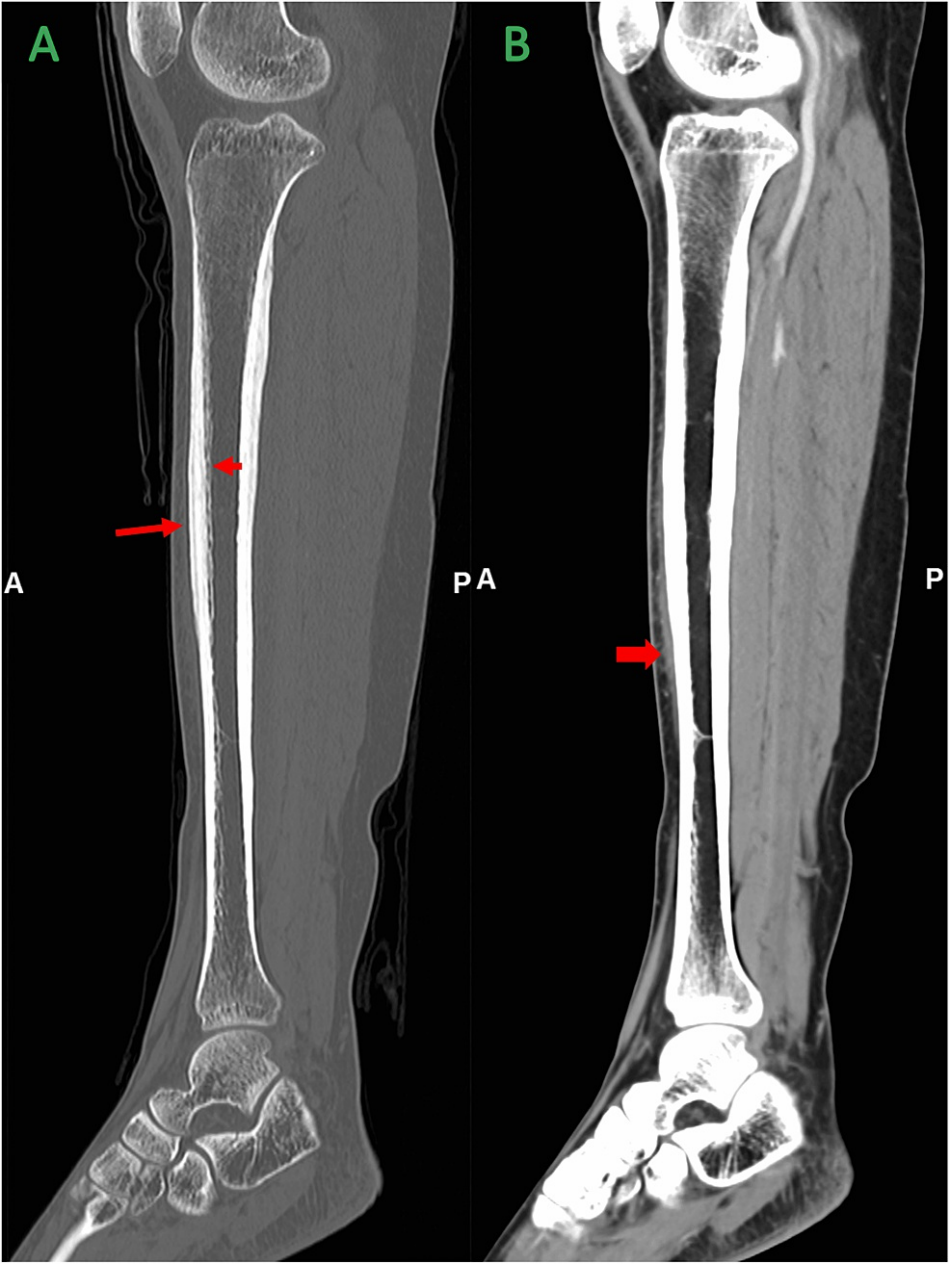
Sagittal CT images of right tibia A: Bone window, shows diaphyseal cortical thickening of the right tibia with solid, thick periosteal reaction (long arrow) and mild narrowing of marrow cavity (short arrow); B: Soft tissue window, shows associated subcutaneous tissue streakiness anteriorly (thick arrow) with no focal collection or intramuscular lesion
